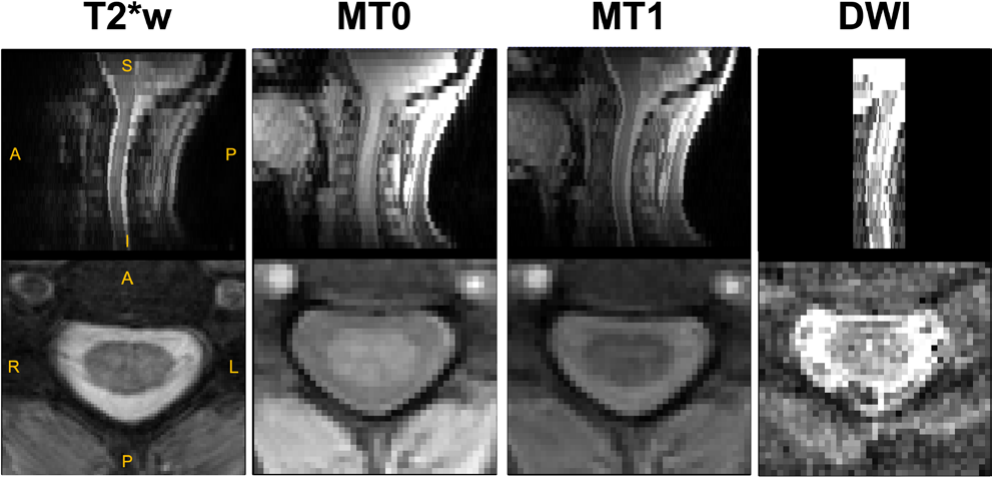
Representative raw data of T2*w, MT0/MT1, and DWI from a single subject.
